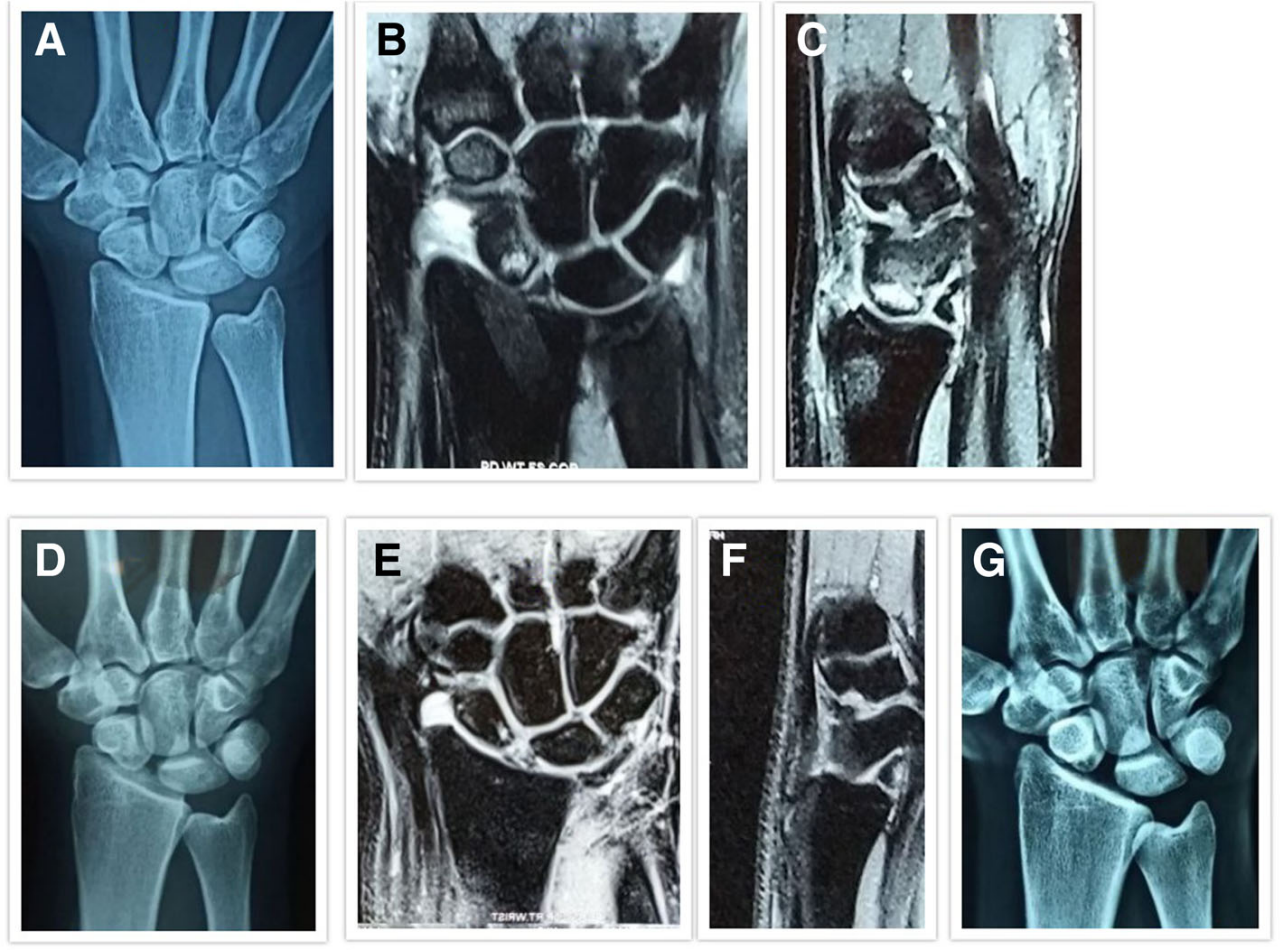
a Pre-treatment anteroposterior radiographs of a 23-year-old male diagnosed with Herbert and Lanzetta stage II Avascular necrosis of the scaphoid. b, c Pre-treatment MRI coronal and sagittal view showing BME. d Post-treatment anteroposterior radiographs at 6 months showing no radiological collapse. e, f Post-treatment MRI coronal and sagittal view at 6 months showing complete resolution of BME. g Post-treatment anteroposterior radiographs at 32 months follow-up showing no radiological collapse/progression with stabilization of the AVN in stage II
